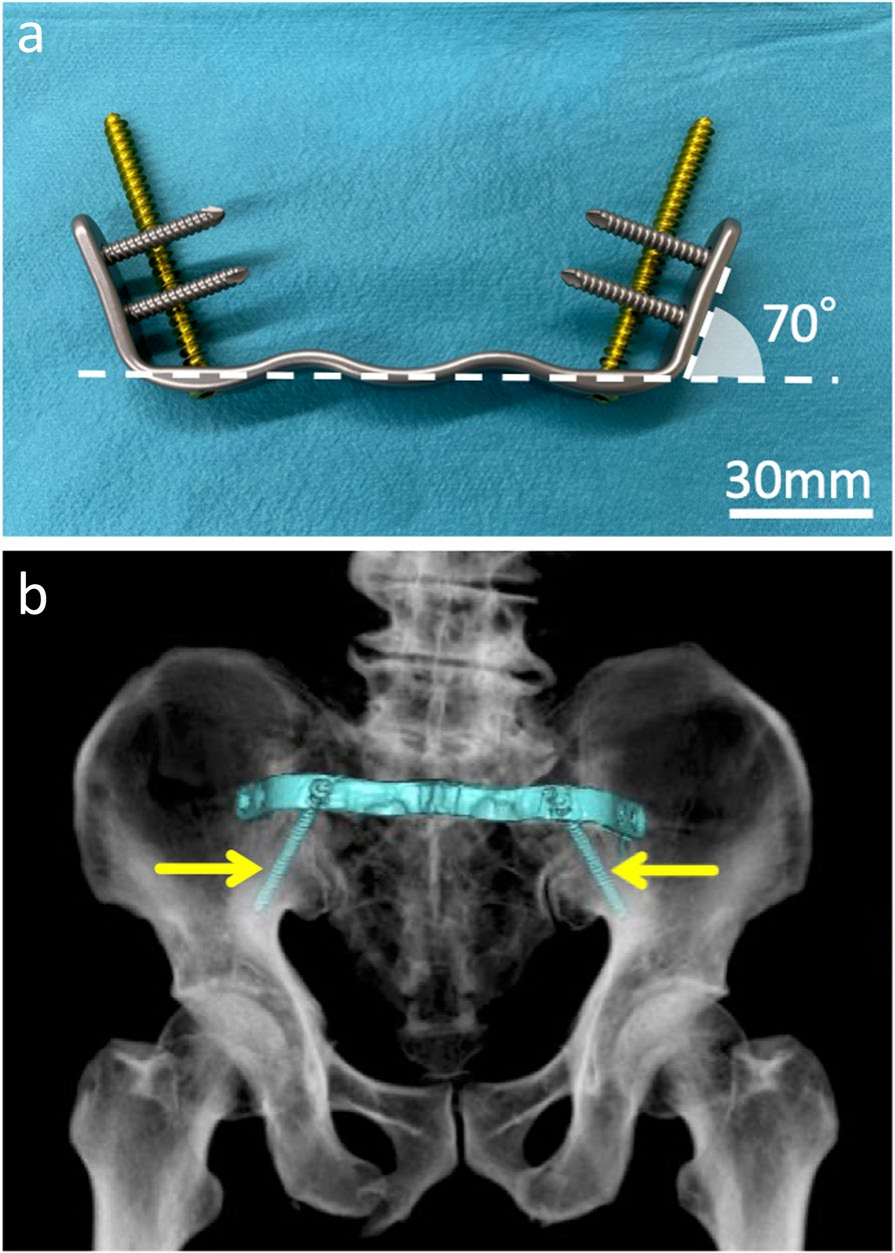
Characteristics of the gull wing plate. a The GWP design takes into account the bilateral ilia, S1 spinal process, and paravertebral muscle. The angle between the posterior surface and the bilateral wings is 70°. Two longer cancellous screws (φ6.5 mm; gold) toward the AIIS and four tri-cortical locking screws (φ5.0 mm; silver) penetrating the iliosacral joint can be inserted. Scale bar: 30 mm. b 3D reconstruction of the postoperative CT scan from the posterior side. Bilateral cancellous bone screws are inserted as far as possible (yellow arrows). GWP, gull wing plate; AIIS, anterior inferior iliac spine; CT, computed tomography
